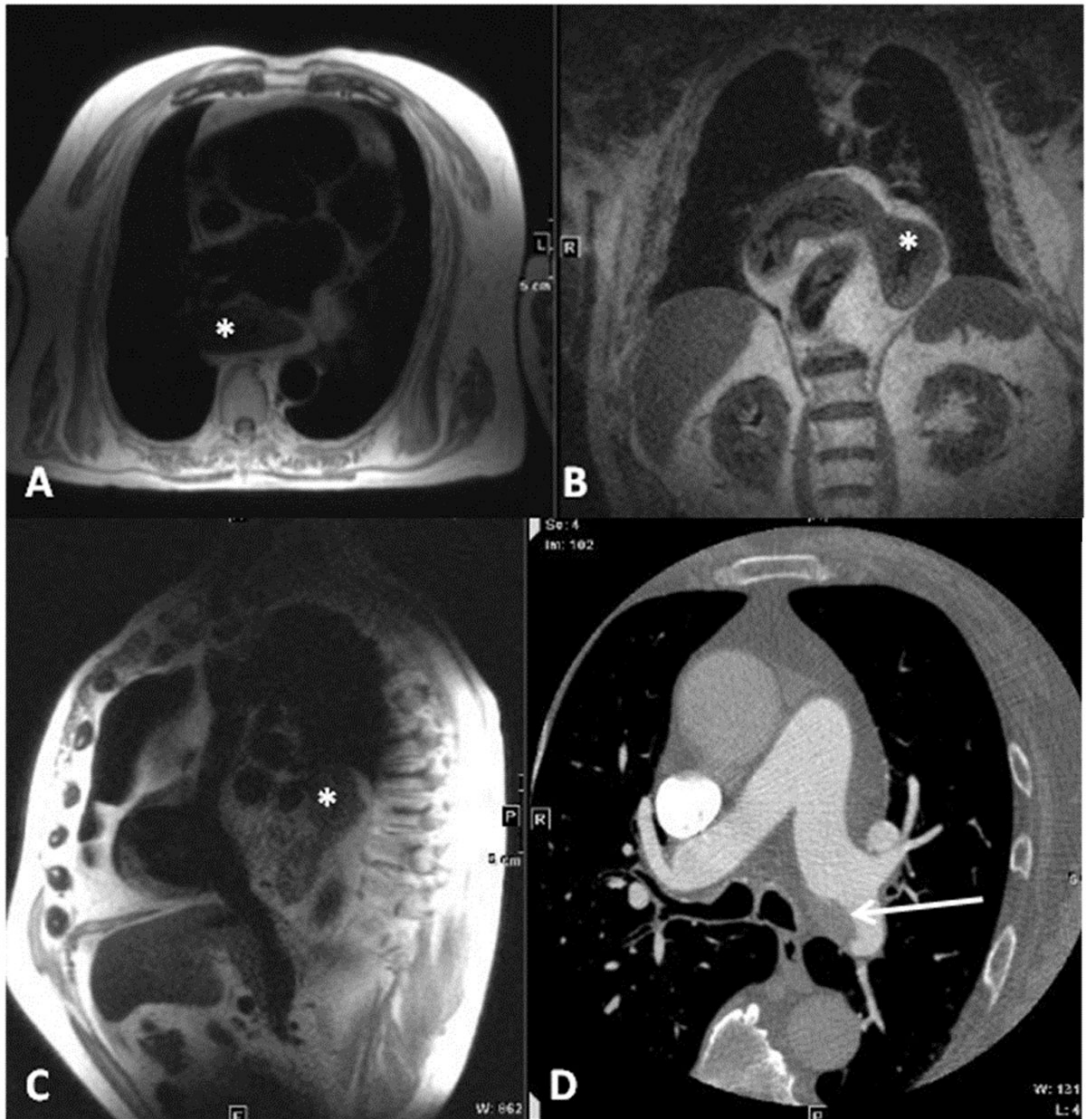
Gastric herniation and angiosarcoma primarily found at CMRI. In a 77 years old male, CMRI primarily detected a thoracic gastric herniation (white stars, A: transversal, Haste, TR 800, TE 46; B: coronal, Haste, TR 800, TE 46; and C: parasagittal, Haste, TR 800, TE 46). An angiosarcoma of the left pulmonary artery was seen in a contrast medium enhanced follow-up thoracic CT scan in the same patient (D: white arrow, axial, 64-slices CT). Recommendation for additional diagnostics was given. Findings were defined as "significant".
